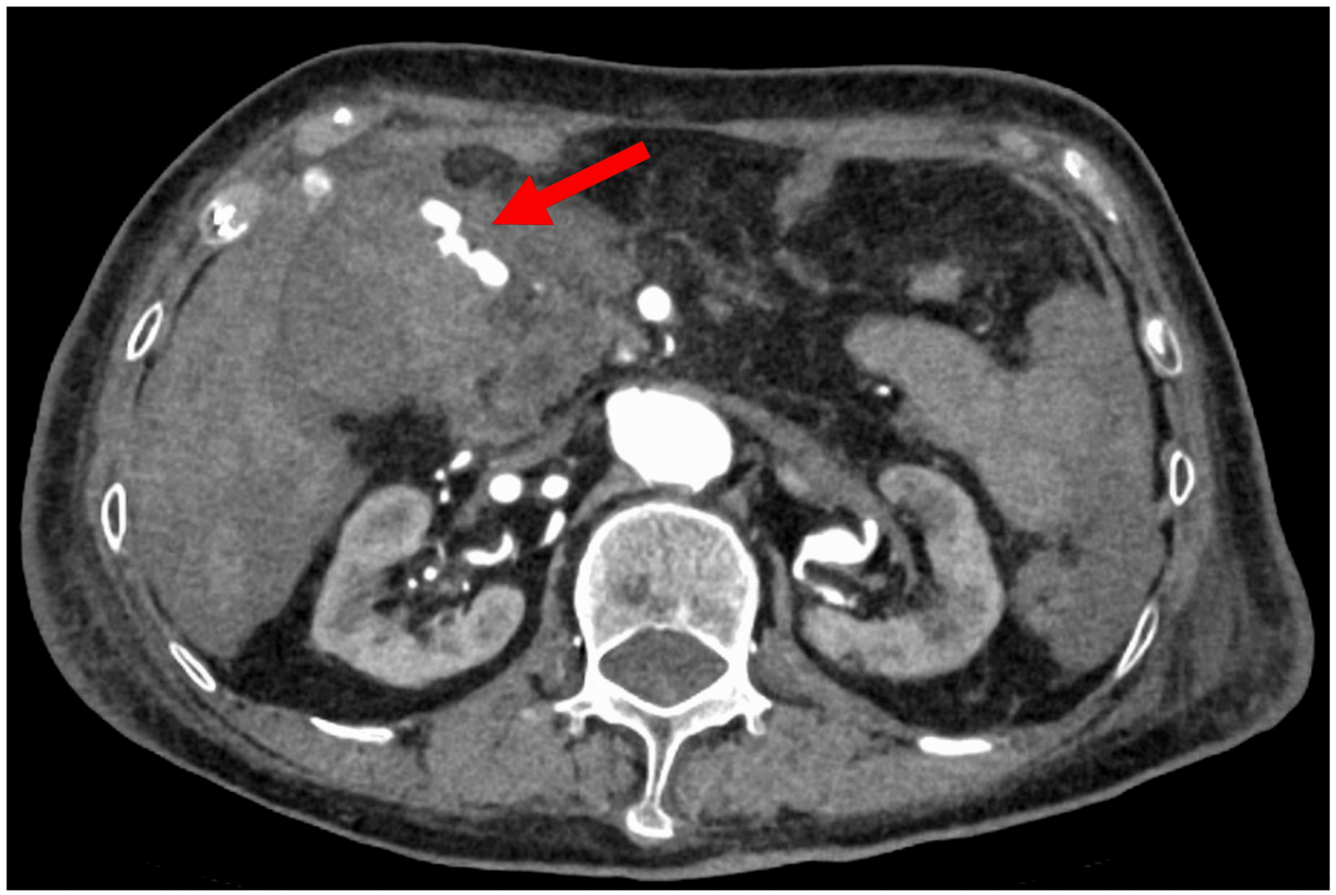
Contrast-enhanced abdominal computed tomography (Case 1) The red arrow indicates active extravasation from the gastroduodenal artery.
